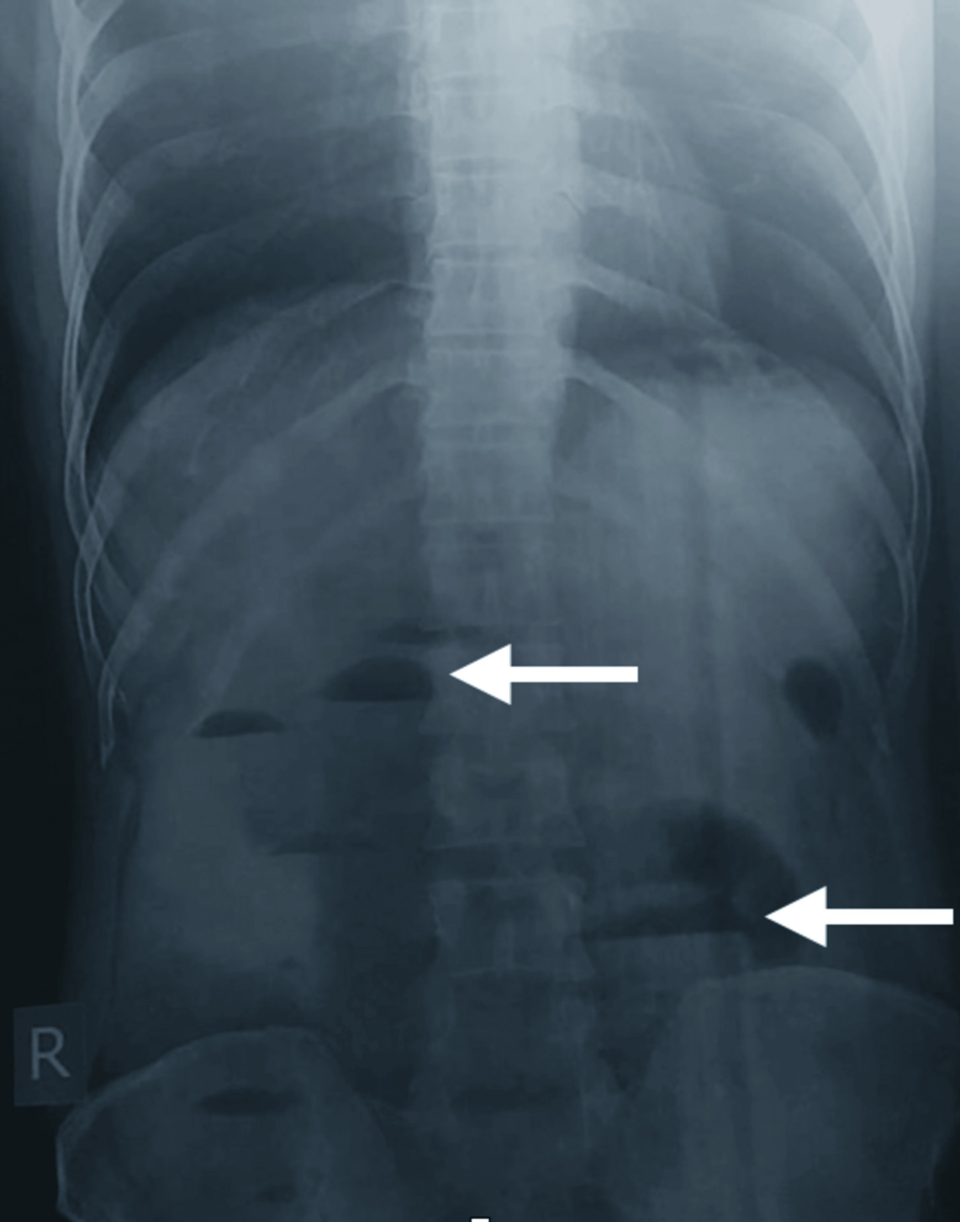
X-ray abdomen erect showing multiple air fluid levels.
